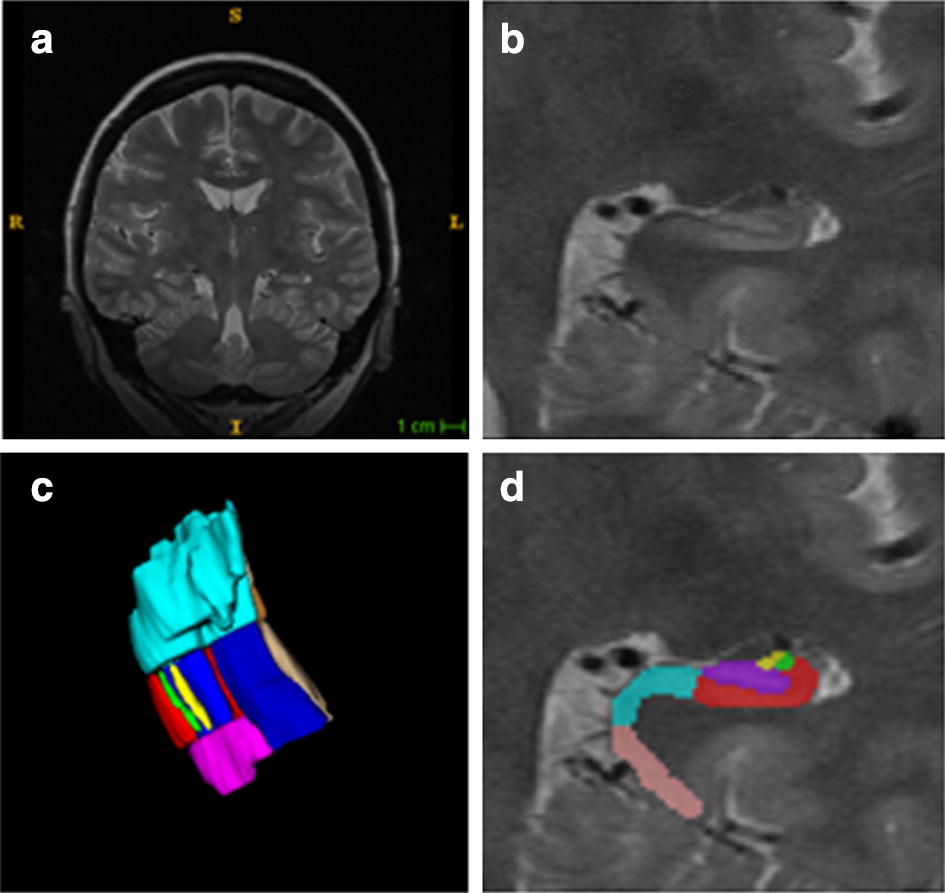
The hippocampus in T2-weighted brain MR image. a The T2-weighted brain image. b The ROI of hippocampus. c The 3D rendering of hippocampal. d The labeled image of hippocampus
